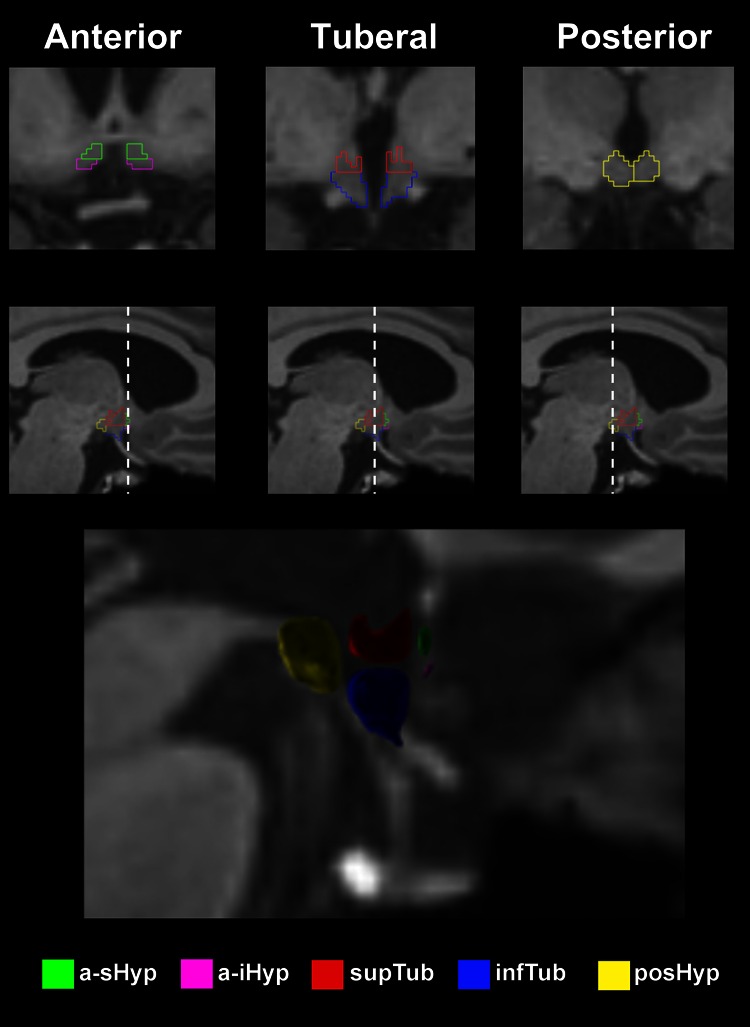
Segmentation of the hypothalamic subunits mapped on a 3T T1-weighted MR image of a control subject and their 3D reconstruction on a sagittal view. *a-sHyp* anterior superior hypothalamus, *a-iHyp* anterior inferior hypothalamus, *supTub* superior tuberal hypothalamus, *infTub* inferior tuberal hypothalamus, *posHyp* posterior hypothalamus
